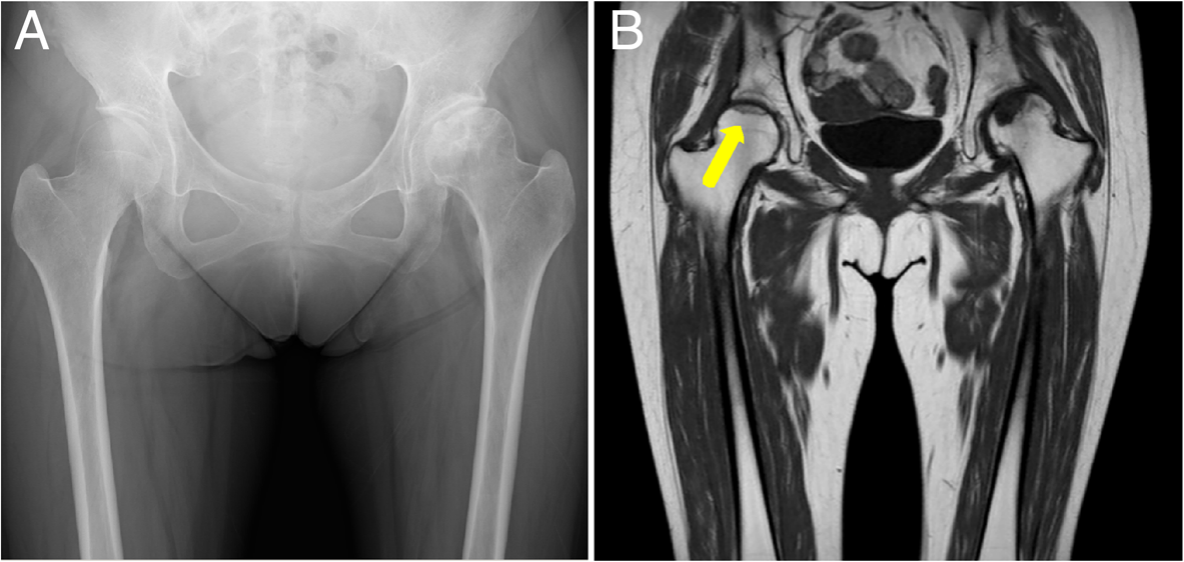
Images obtained at 1 year after corticosteroid dose increase. (a) Anteroposterior (AP) radiograph of the hip 1 year after corticosteroid dose increase. (b) Coronal T1-weighted magnetic resonance image of the hip in the same month of the AP radiograph. Low-intensity band pattern in the right femoral head (arrow)
